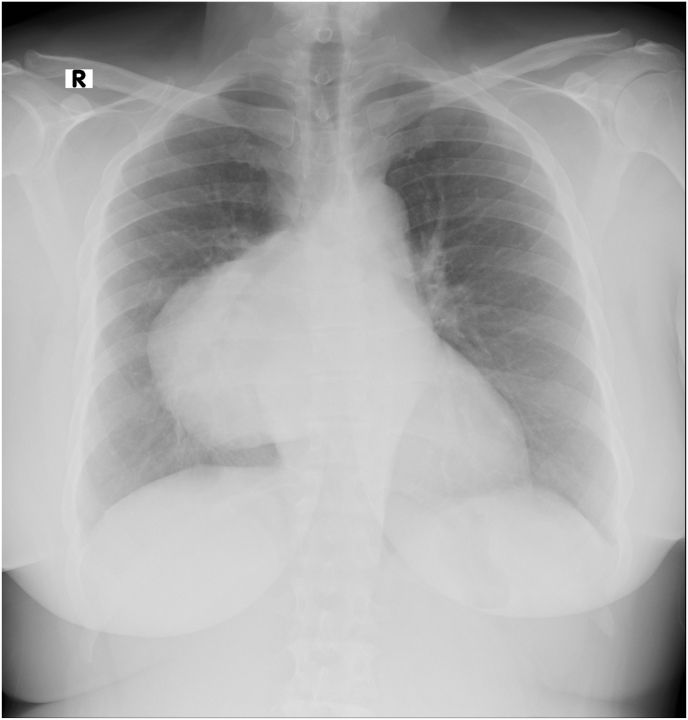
Chest X-Ray showing mass in right hemithorax.
